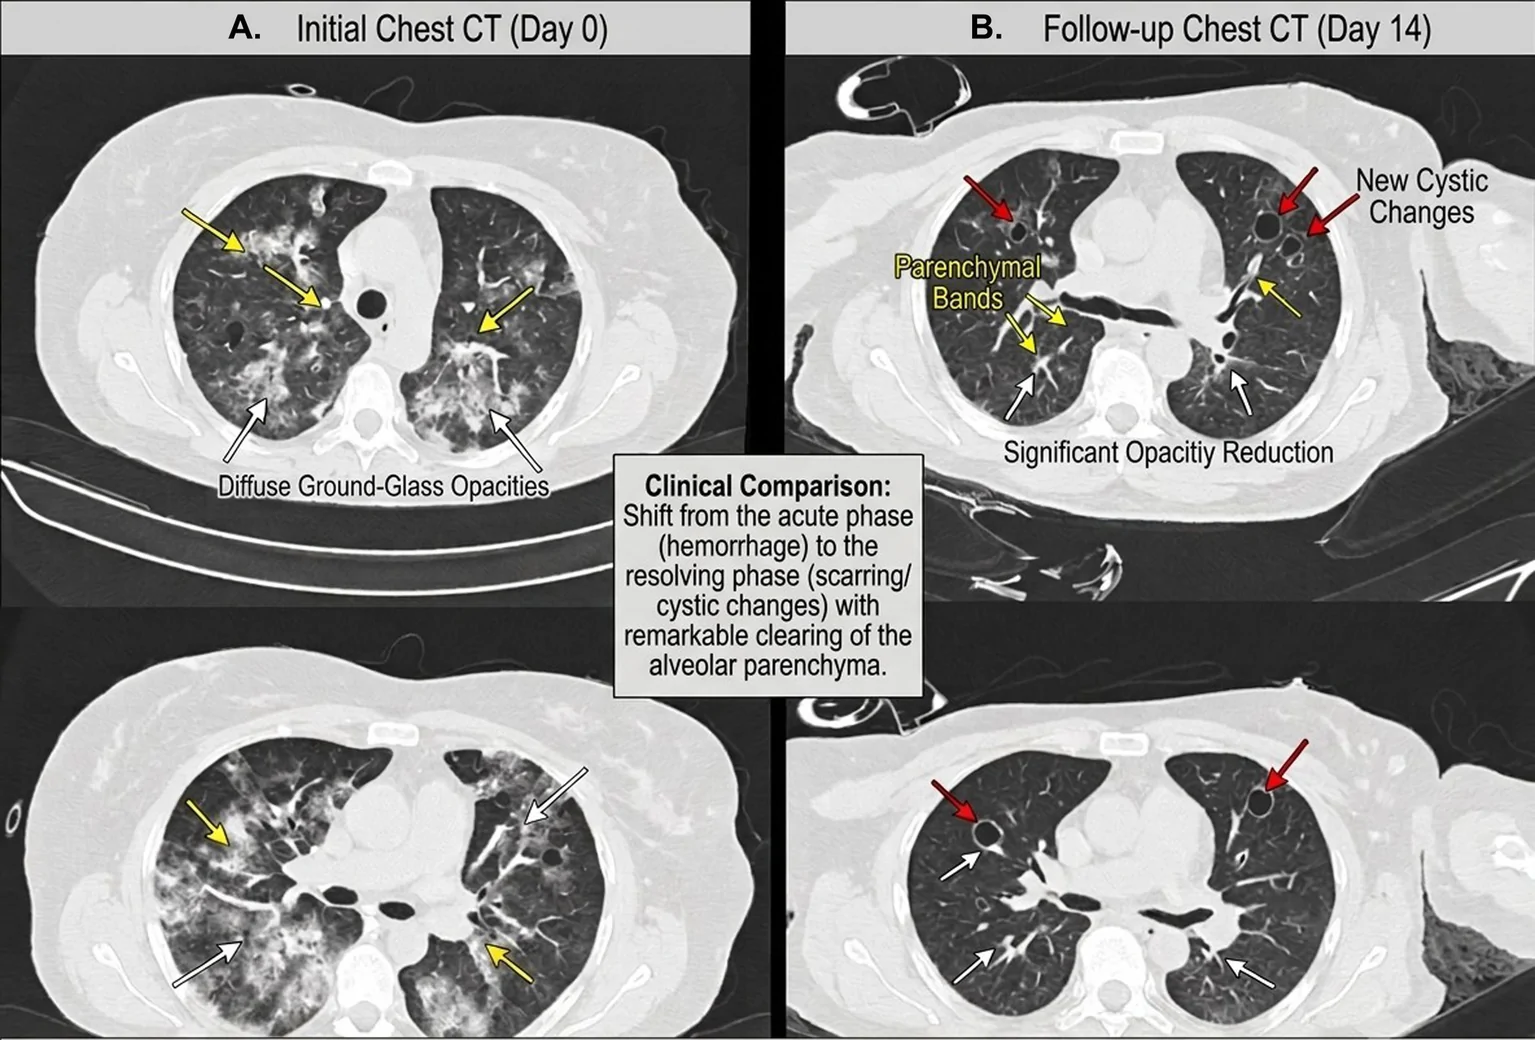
Non-contrast chest computed tomography (CT) scan—admission and control. (A) (Day 0): Chest CT showing diffuse ground-glass opacities and areas of hemorrhage (white). The scan shows extensive lung involvement. (B) (Day 14) Follow-up CT demonstrating dramatic interval reduction of ground-glass opacities. There are new findings of persistent parenchymal bands (yellow arrow) and development of pulmonary cysts (red arrows), indicating a shift from hemorrhage to scarring/organizing phase.
